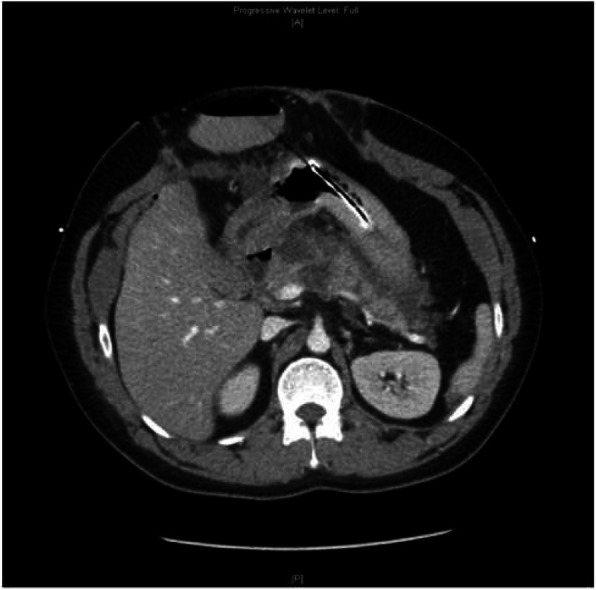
Computed tomography of the abdomen a week after surgical decompression revealed heterogeneous enhancement in the pancreatic head and body consistent with necrosis. An immature collection is starting to form adjacent to the pancreatic tail. Axial view
